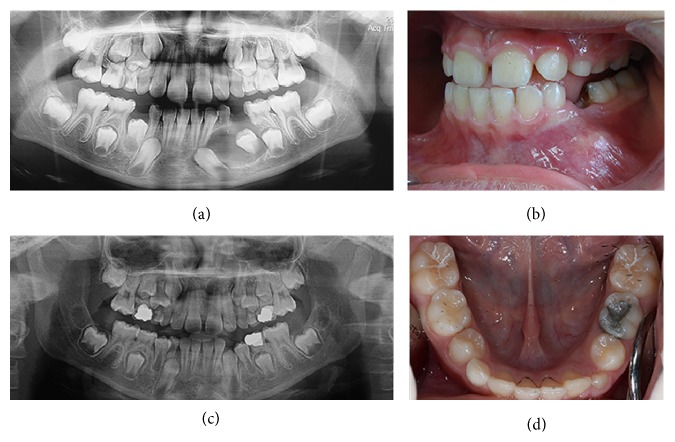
Case 3. (a) Initial panoramic X-ray image showing a DC in the left mandibular region. (b) Initial intraoral view of the cystic region. (c)-(d) 12-month follow-up X-ray image and intraoral photo showing the complete healing of the cystic region and eruption of the affected tooth.
